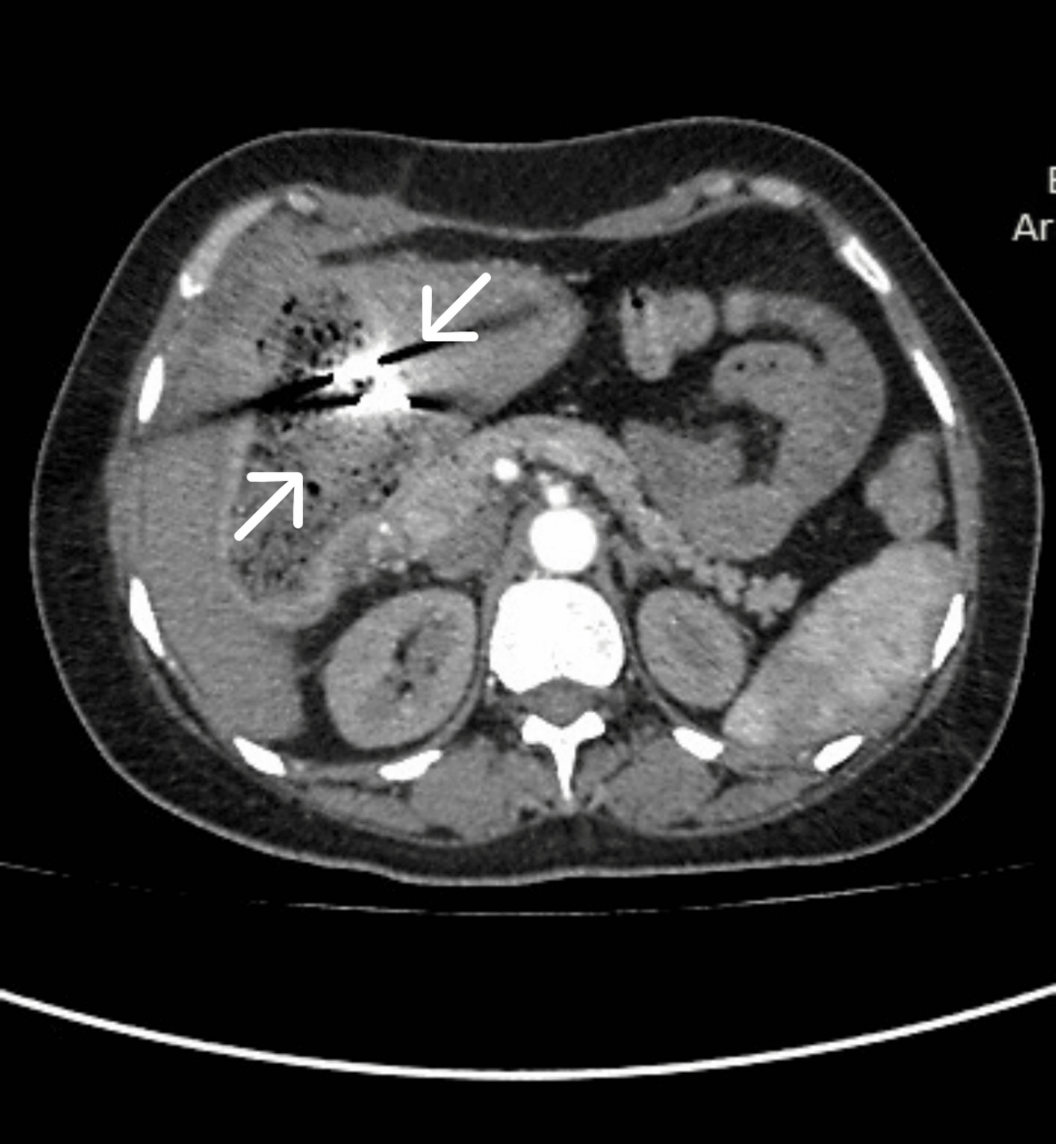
CT (axial view) of the abdomen and pelvis showing the retained surgical pack (arrows)
